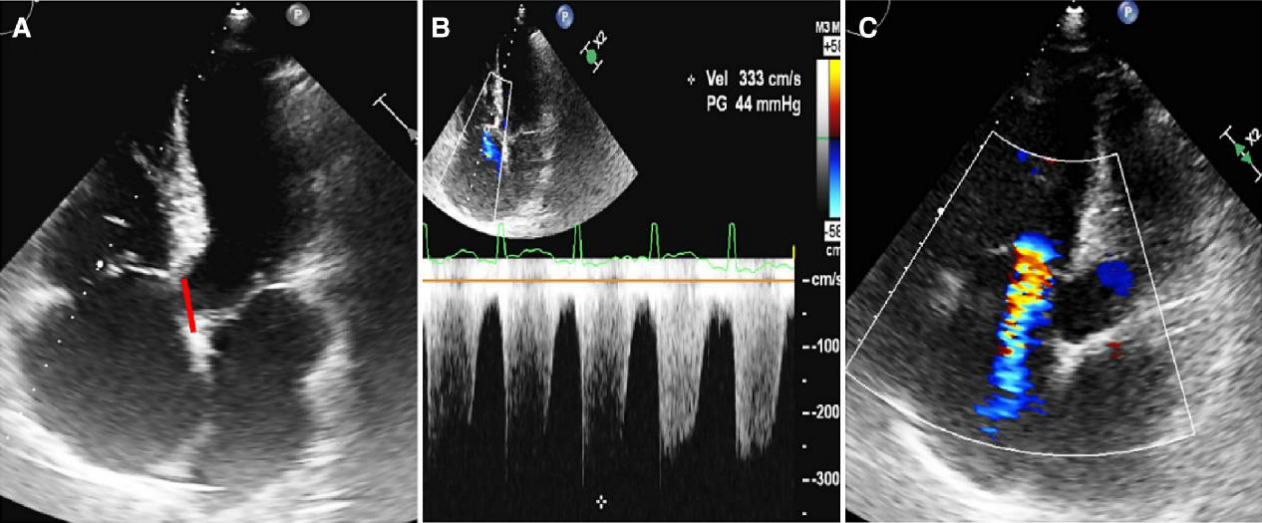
(*A*) Apical 4-chamber view revealing apical displacement of septal leaflet of the tricuspid (straight line) with (*B*) moderate TR. (*C*) Colour Doppler exhibiting tricuspid regurgitation with a jet velocity of 3.33 m/s and a maximum gradient of 44 mmHg. A4C, apical 4 chamber; TR, tricuspid regurgitation.
